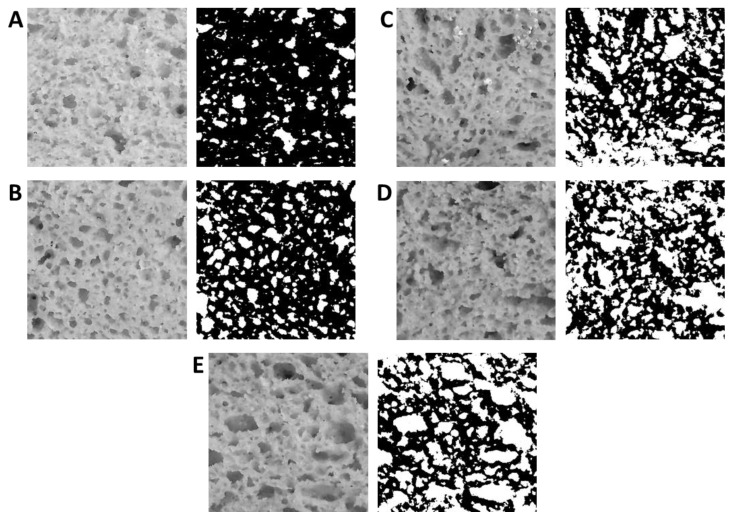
Representative images including digital images of bread showing the original gray-level images and computed binary results from gray-level thresholding at the two-cluster of bread crumb. b-CT (A), bread made with rice and corn flours (ratio 1:1) and 6% (*wt*/*wt*) of structuring flours (psyllium, hydrated chia, and flaxseed, ratio 4:1:1); b-S (B), bread made with rice and corn flours (ratio 1:1), 30% (*wt*/*wt*) of s-C20, and 6% of structuring flours (psyllium, hydrated chia, and flaxseed, ratio 4:1:1); b-ST (C), bread made with rice and corn flours (ratio 1:1), 30% (*wt*/*wt*) of s-C20, 10% (*wt*/*wt*) of teff flour, and 6% of structuring flours (psyllium, hydrated chia, and flaxseed, ratio 4:1:1); b-SL (D), bread made with rice and corn flours (ratio 1:1), 30% (*wt*/*wt*) of s-C20, 10% (*wt*/*wt*) of red lentil flour, and 6% of structuring flours (psyllium, hydrated chia, and flaxseed, ratio 4:1:1); b-SQ (E), bread made with rice and corn flours (ratio 1:1), 30% (*wt*/*wt*) of s-C20, 10% (*wt*/*wt*) of quinoa flour, and 6% of structuring flours (psyllium, hydrated chia, and flaxseed, ratio 4:1:1). All doughs had DY of 200. Baker’s yeast was used at 1.25% (*wt*/*wt*). Proofing was at 30 °C for 2 h and baking at 200 °C for 45 min.
